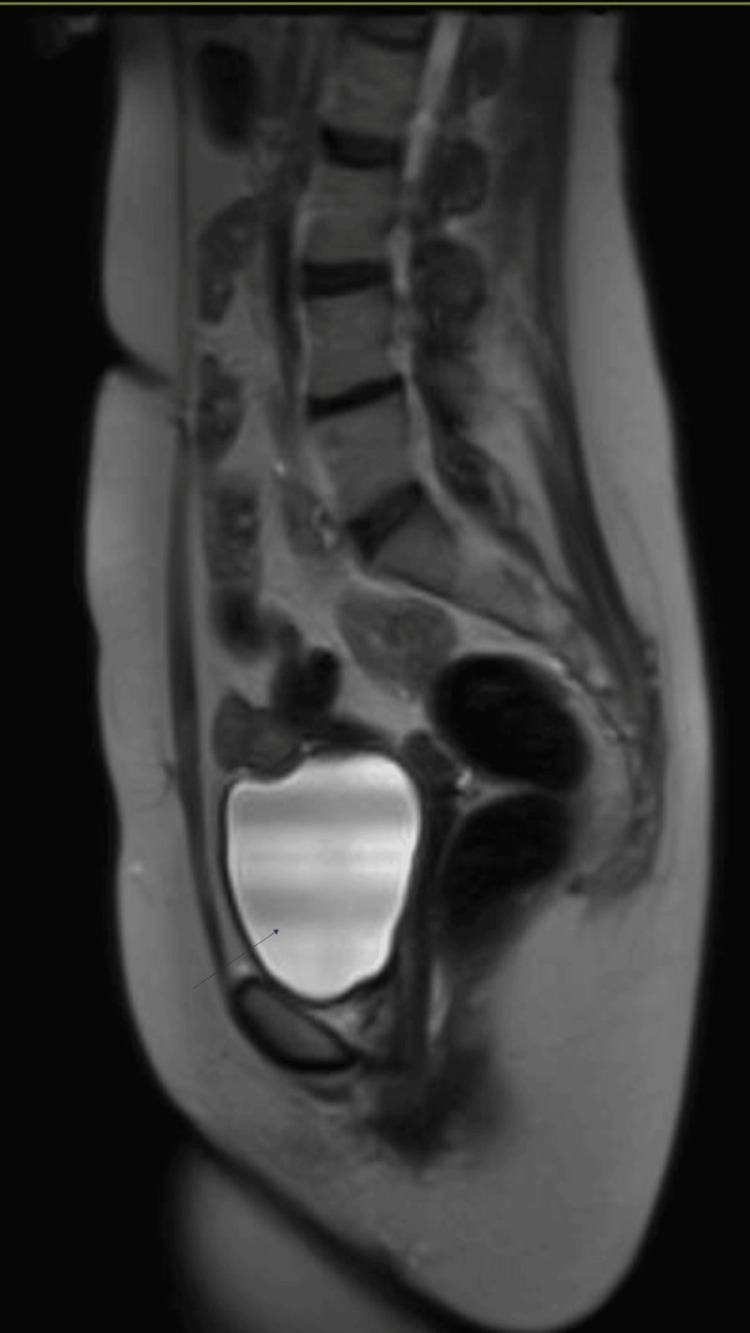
MRI sagittal plane: Heterogenous cystic mass in left adnexa, adjacent to vaginal vault consistent with dermoid cyst as evidenced by small area of lipid content within the lesion. Hyperintense area seen on T2-weighted image.
